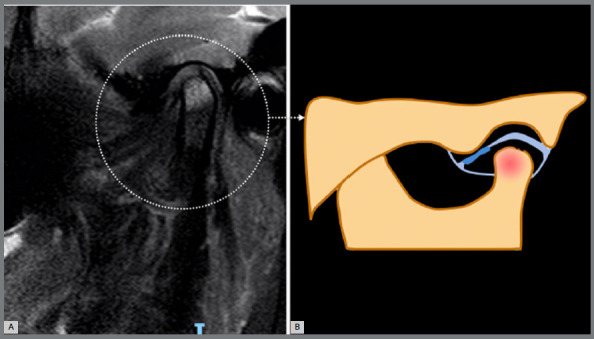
Bone phenotype: a bone marrow lesion in the condyle, and an irregularly condylar head and/or articular fossa contour. A) Referential temporomandibular joint MRI sagittal T2-weighted image. B) Temporomandibular joint scheme. Adapted from ROAMES - Rapid OsteoArthritis MRI Eligibility Score (Source: Roemer et al.^8^, 2020).
